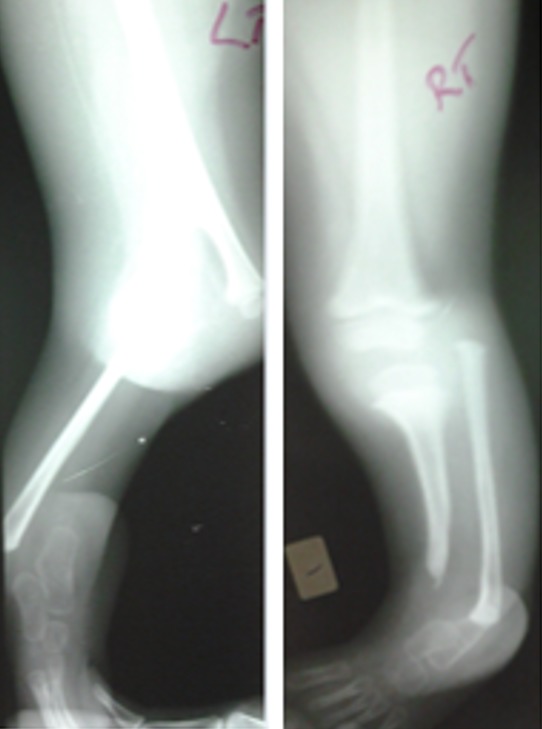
Right and left radiographs of femur and tibia/fibula
